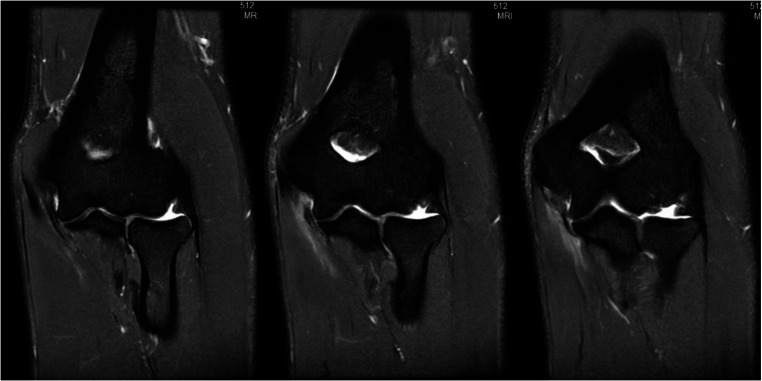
Coronal proton density weighted MRI series demonstrating a partial proximal tear of the ulnar collateral ligament
